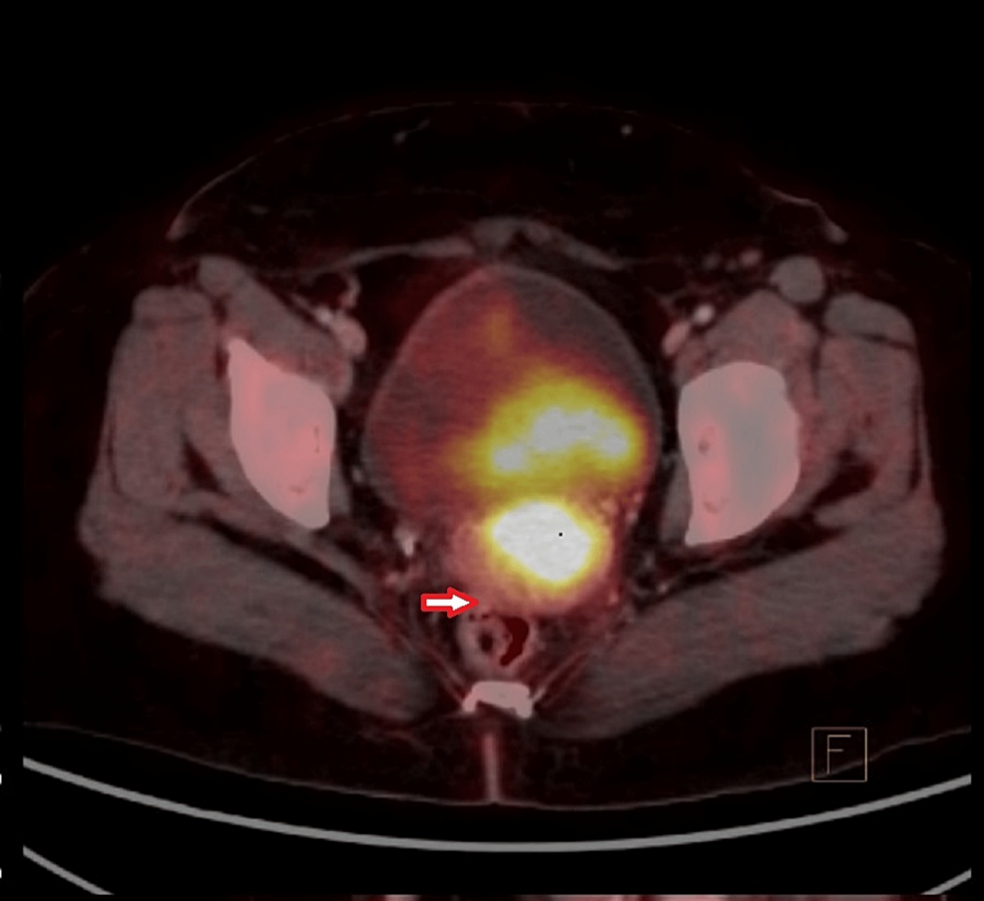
The PET/CT image showing involvement of parametrium The red arrow shows the extension of FDG uptake beyond the cervix. PET/CT: Positron emission tomography/computed tomography, FDG: Fluoro- D-glucose
